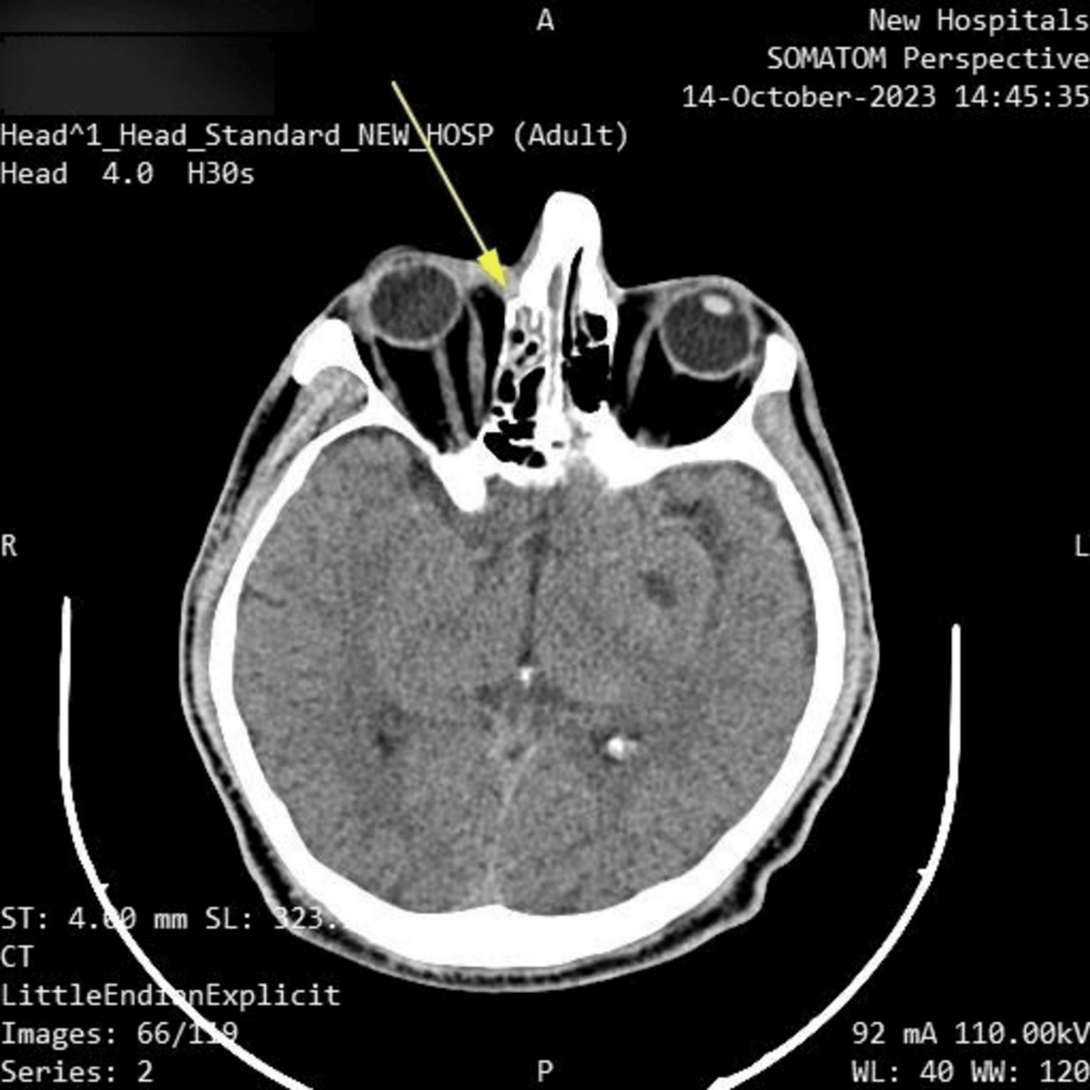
Axial CT head Right ethmoidal sinusitis (yellow arrow)
